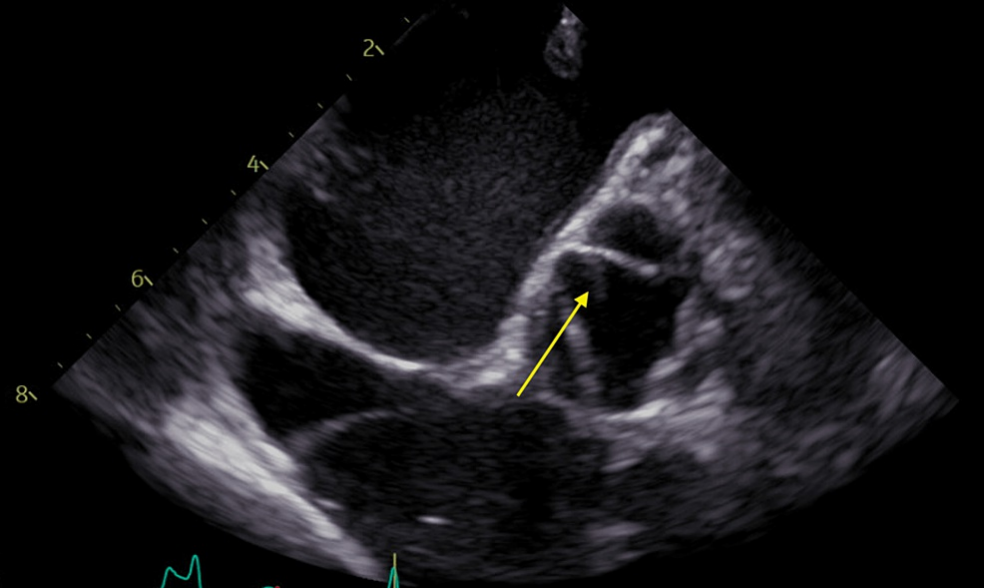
Transesophageal echocardiogram showing fine filamentous strand (yellow arrow) on the aortic valve
